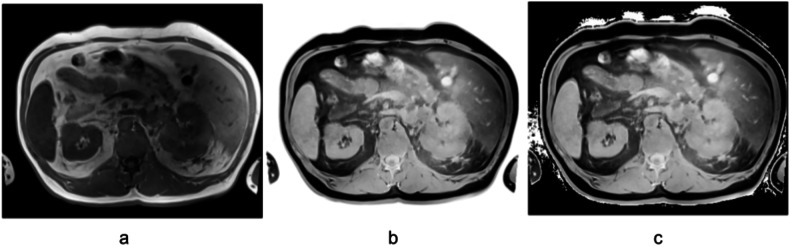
a Unprocessed T1-weighted image, (b) inverted image, and (c) inverted + black background image
